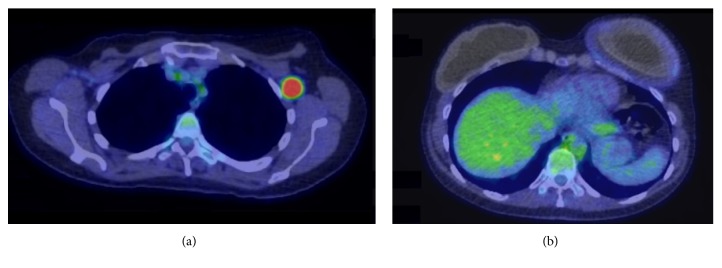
PET-CT scan images. (a) Pathologically enlarged, metabolically active left axillary lymph node. (b) Metabolically active thickening and seroma around left breast implant.
